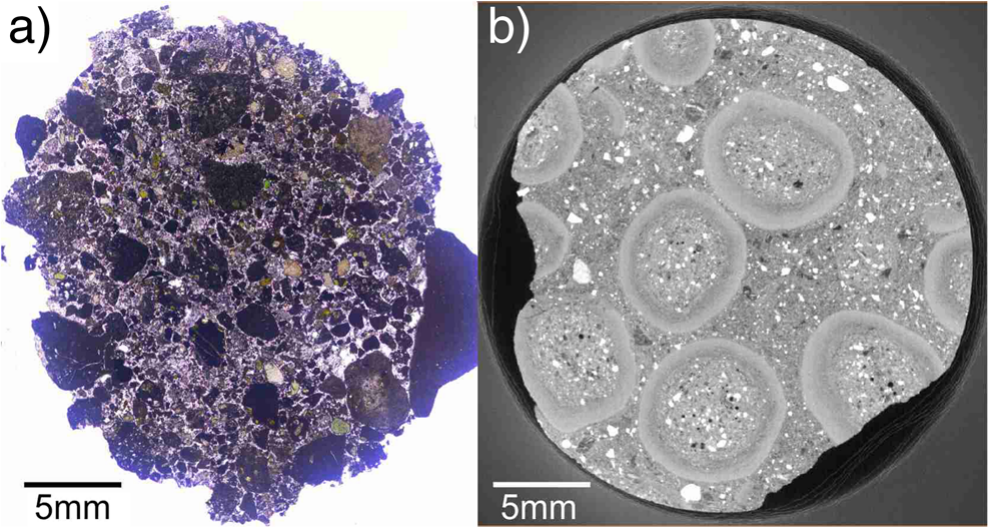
Examples of aggregates containing abundant coarse clasts. a Cape Verde particle cluster (PC) with primary particle sizes of up to several mm. b Drill core from Caldera del Rey, Tenerife, analyzed with X-ray CT. AP2 type aggregates are embedded in matrix material. AP2 aggregates have coarse-grained cores and fine-grained rims; PSD of aggregates does not exceed maxima of 500 μm. Matrix material lacks fine-grained ash as it is bound in the rims of the aggregates, and PSD does not exceed maxima of 1.5 mm
